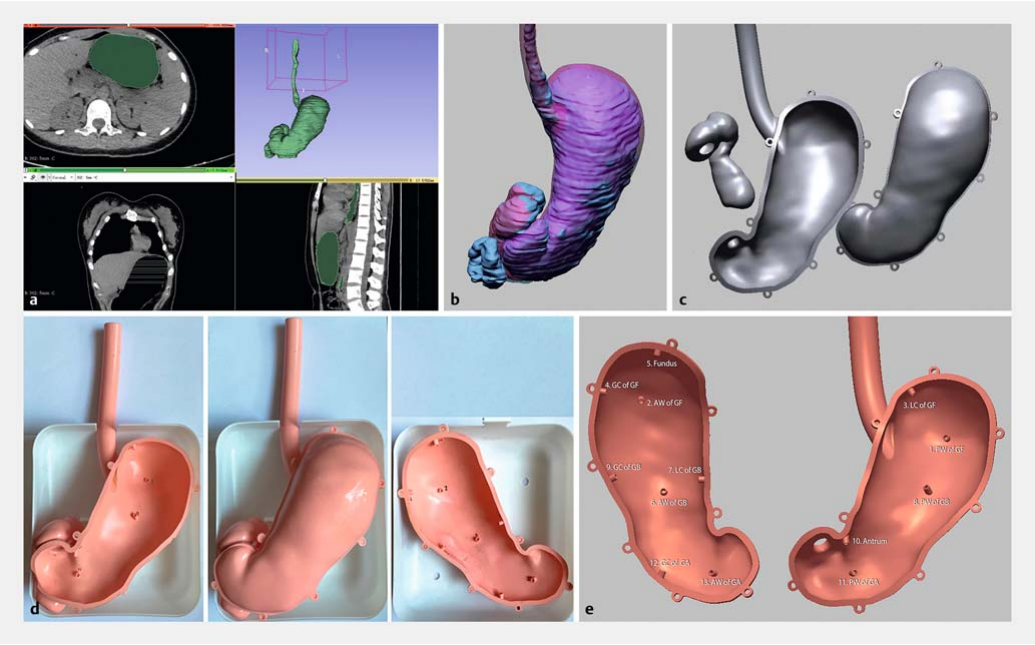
CT imaging produces a basic gastric morphological structure.
